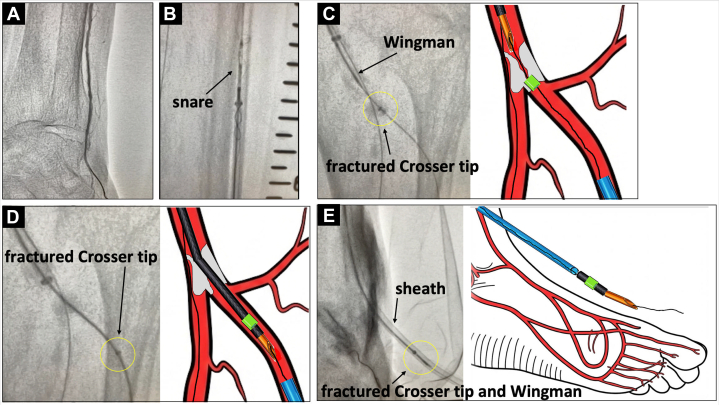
Pull-Through Rail Creation and Push-and-Release Maneuver (A) Ultrasound-guided retrograde dorsalis pedis access and sheath placement. (B) A snare advanced from the retrograde sheath captures and externalizes the antegrade guidewire, creating a through-and-through rail (Video 2). (C) A bevel-tipped Wingman catheter is advanced antegradely along the rail to the proximal edge of the entrapped fragment. (D) Short, coaxial pushes with the Wingman dislodge the fragment from the calcified shelf (Video 3). (E) The freed fragment is pushed into the retrograde sheath and removed en bloc (Video 4).Step 3: Create the Rail (Crucial Safety Maneuver). A standard pull-through technique often involves passing a new retrograde wire. However, to avoid the risk of losing the track through the fragment, we used the existing antegrade wire. We advanced a snare from the retrograde pedal sheath up to the anterior tibial artery (Figure 2B). We successfully captured the tip of the antegrade guidewire (which was still holding the fragment) and externalized it through the pedal sheath. This created a stable through-and-through rail using the original workhorse wire, ensuring continuous control of the fragment without the risk of wire exchange (Video 2).Step 4: Coaxial “Push and Release.” We advanced a bevel-tipped supportive Wingman catheter (ReFlow Medical) antegradely over the tensioned rail until it abutted the proximal end of the fragment (Figure 2C). Instead of pulling (which had caused the wedge effect), we applied short, coaxial pushes with the Wingman catheter. The rail provided the necessary support, and the push force successfully dislodged the fragment from the calcified shelf (Figure 2D, Video 3).Step 5: Retrograde Retrieval. The dislodged fragment was pushed distally into the retrograde sheath by advancing the Wingman catheter (Figure 2E), then guided out of the body and retrieved as a single mass (Figure 3, Video 4). Final angiography demonstrated restored blood flow to the dorsal metatarsal and plantar arteries (Figure 4).
